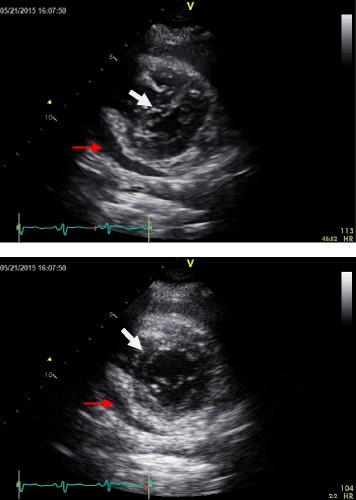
Parasternal short axis still frames of echocardiogram from patient demonstrating small pericardial effusion (red arrows) with ventricular interdependence for diastolic filling as evidenced by a septal shift to the left during right ventricular filling (upper panel, white arrow) and septal shift to the right during left ventricular filling (lower panel, white arrow). In the setting of shock and pericardial effusion, septal bounce is pathognomonic for effusive-constrictive pericarditis with cardiac tamponade
